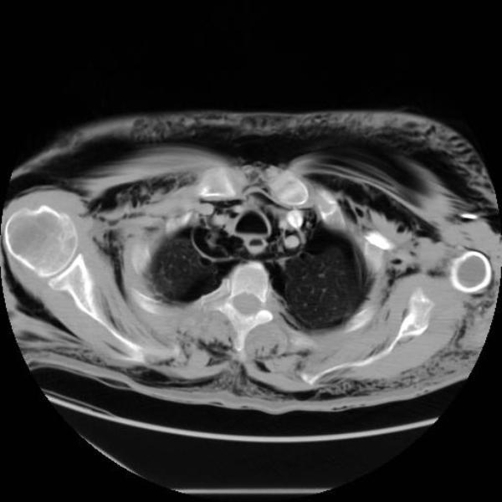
CT showing rib fractures.
